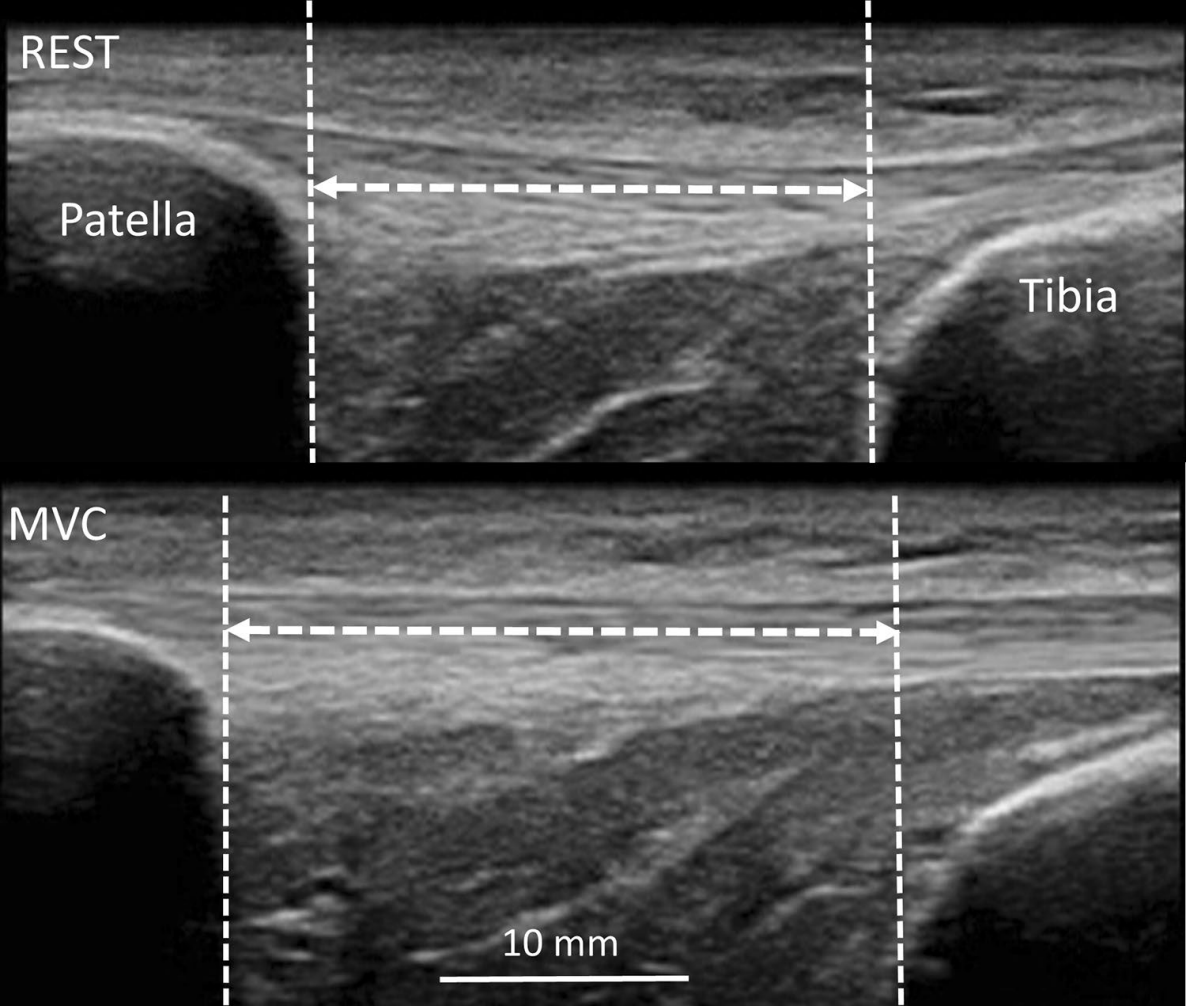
Measurement of patellar tendon elongation on the ultrasound images recorded during one maximal voluntary isometric contraction (MVC)
